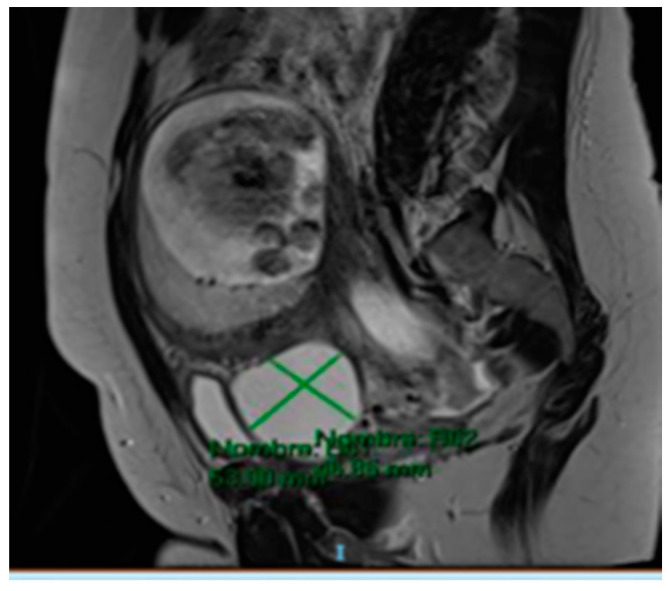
MRI scan compatible with 53 mm × 46 mm cystic formation in the right paracervical area.
